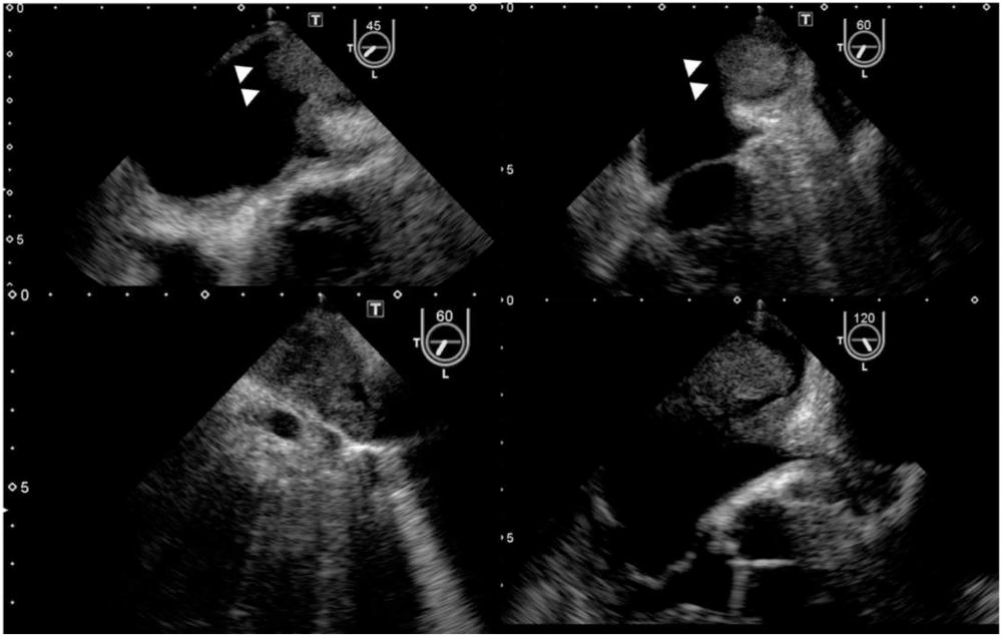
Transoesophageal echocardiography demonstrates a heterogeneous 40 × 25 mm mass obstructing the left pulmonary vein, with partial protrusion into the left atrial cavity (white arrows).
